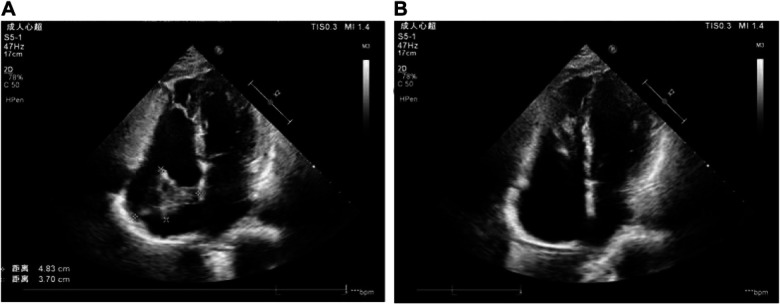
Echocardiography (August 6, 2025). (A) Apical four-chamber view; (B) Apical four-chamber view (supplemental view).
